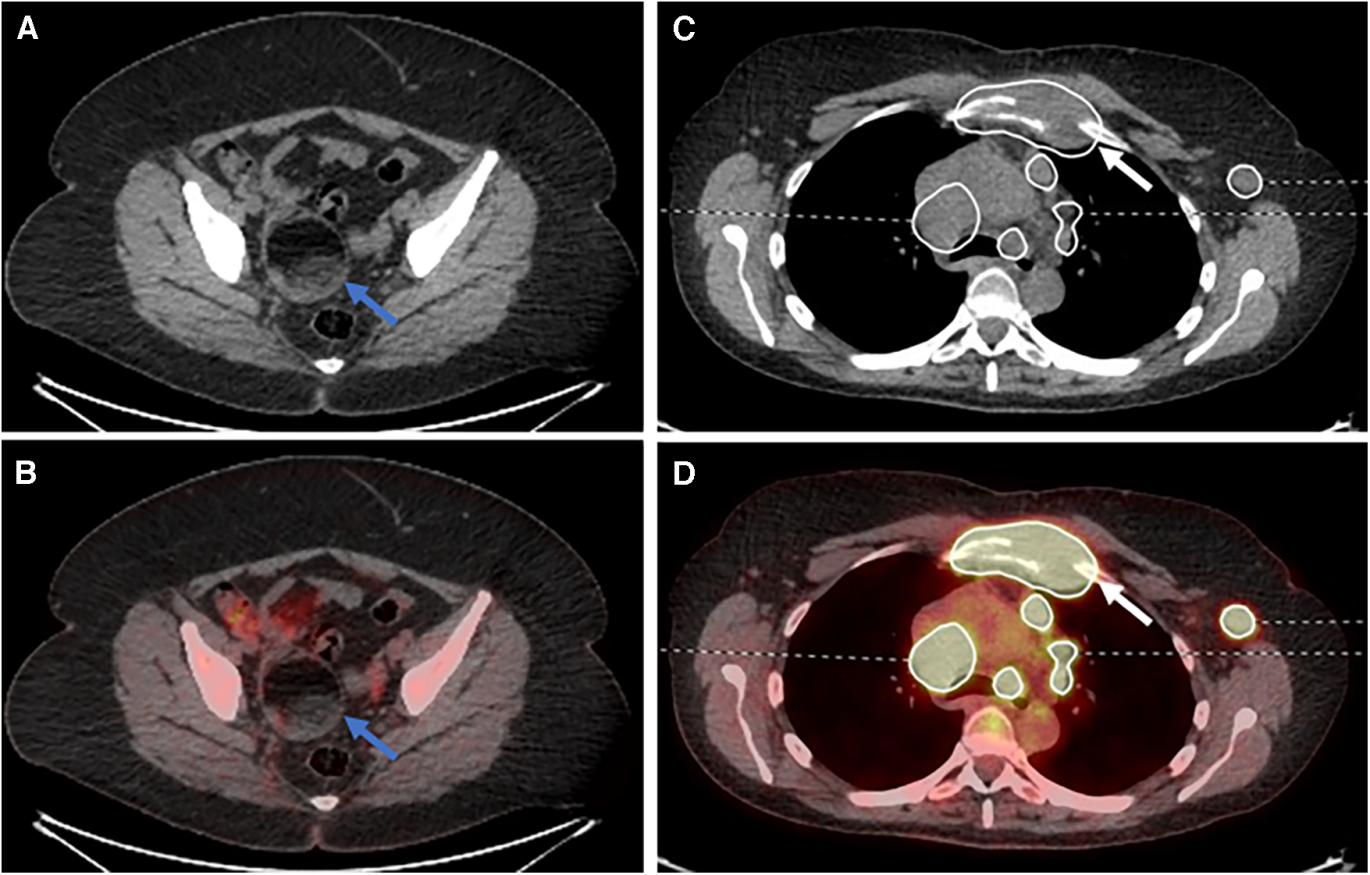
Select (A) axial CT and (B) fused PET/CT images demonstrating an ovarian dermoid cyst (blue arrows). Select (C) axial CT and (D) fused PET/CT images demonstrating destruction of the manubrium due to lymphomatous disease (white arrows).
